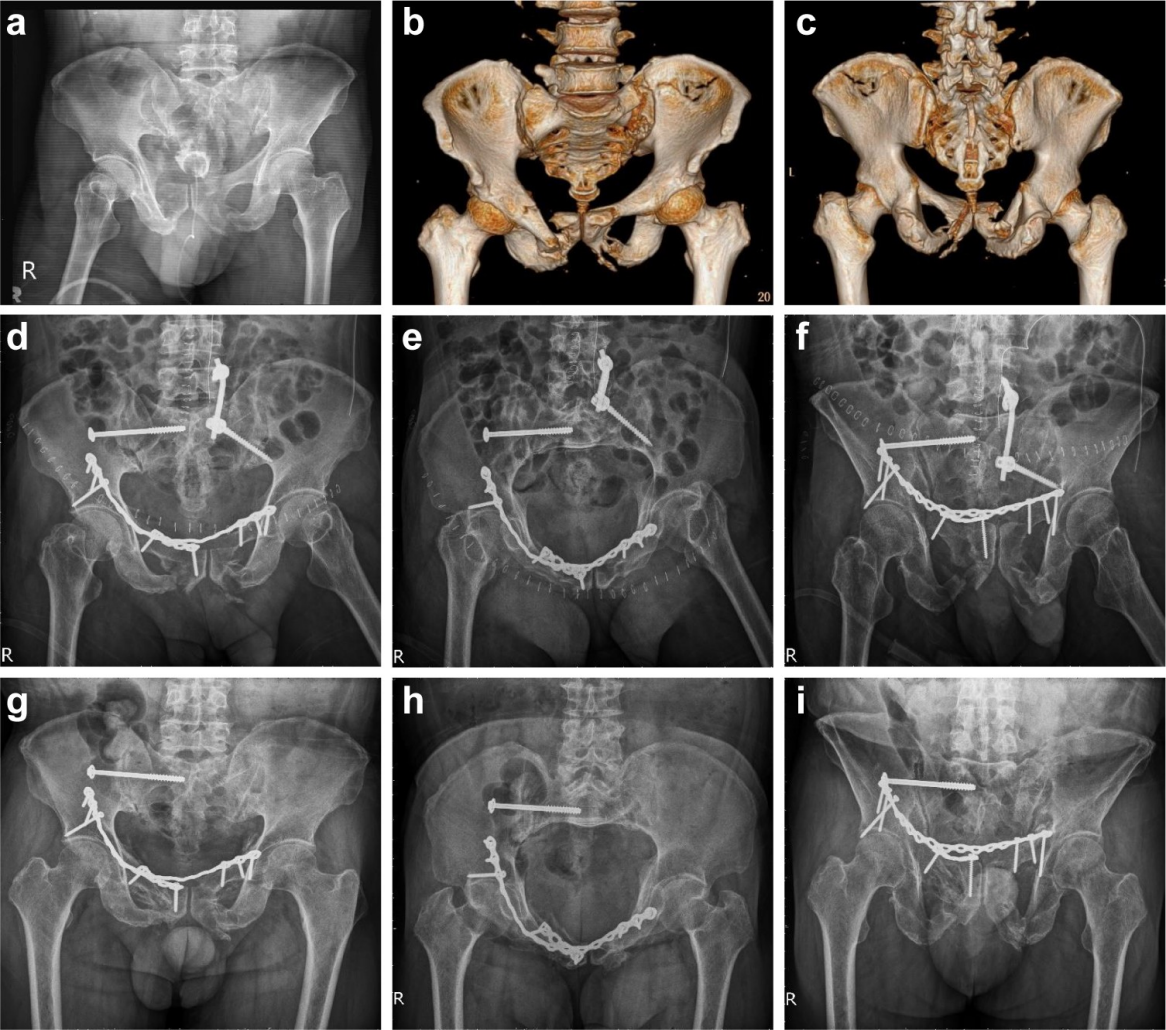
A 62-year-old male with anterior and posterior pelvic ring injury. a Preoperative pelvic anterior-posterior (AP) view. b Preoperative 3D reconstructed computed tomography (CT) anterior view. c Preoperative 3D reconstructed CT posterior view showing sacral fracture with bilateral superior and inferior pubic rami fracture. d, e, f Postoperative pelvic radiology series (AP, inlet, outlet view) demonstrating percutaneous iliosacral screw, spinopelvic fixation of the posterior pelvic ring, and conventional plate fixation of the anterior pelvic ring. g, h, i Postoperative pelvic radiology series (AP, inlet, outlet view) at 12-month follow-up, demonstrating bone union and removal of spinopelvic fixation. The quality of fracture reduction was graded as good.
